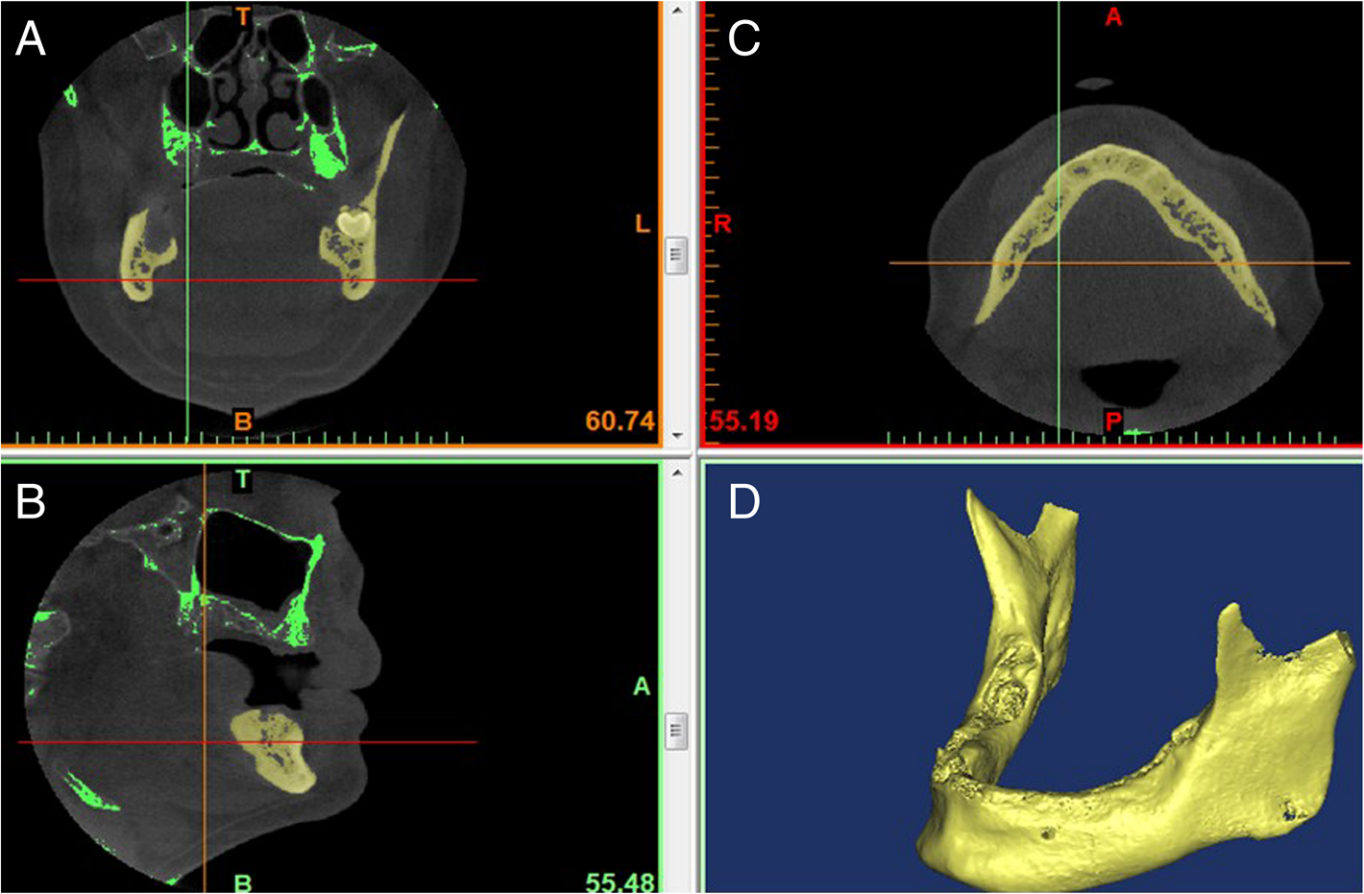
3D virtual model of the mandible reconstructed from CT images. (a) Coronal plane, (b) transverse plane, (c) sagittal plane, and (d) 3D view
